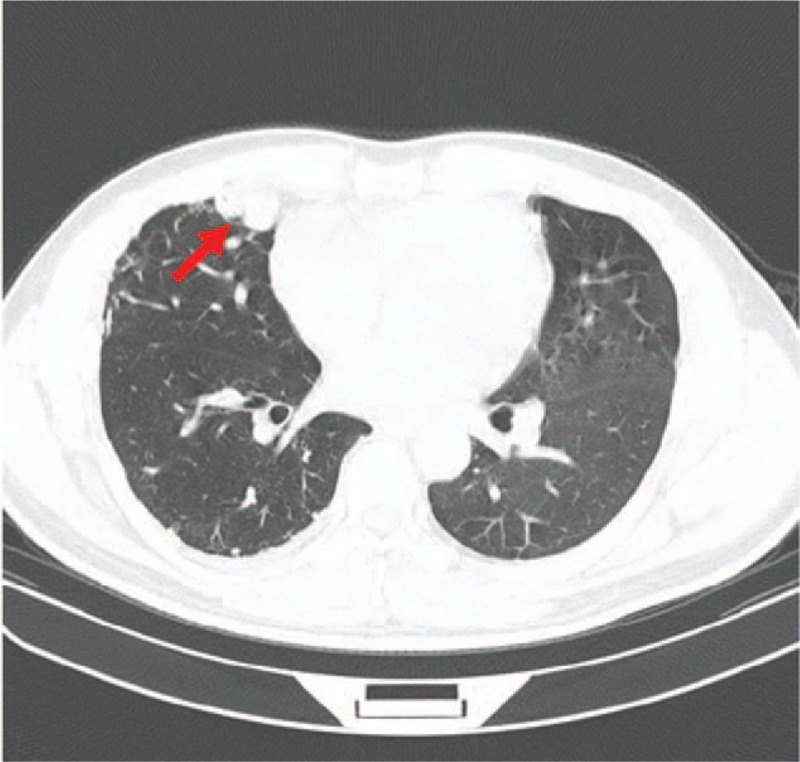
High-resolution CT showing an abnormal vascular mass (red arrow; ∼8.0 × 2.5 mm in size) in the medial segment of the middle lobe of the right lung near the pleura.
